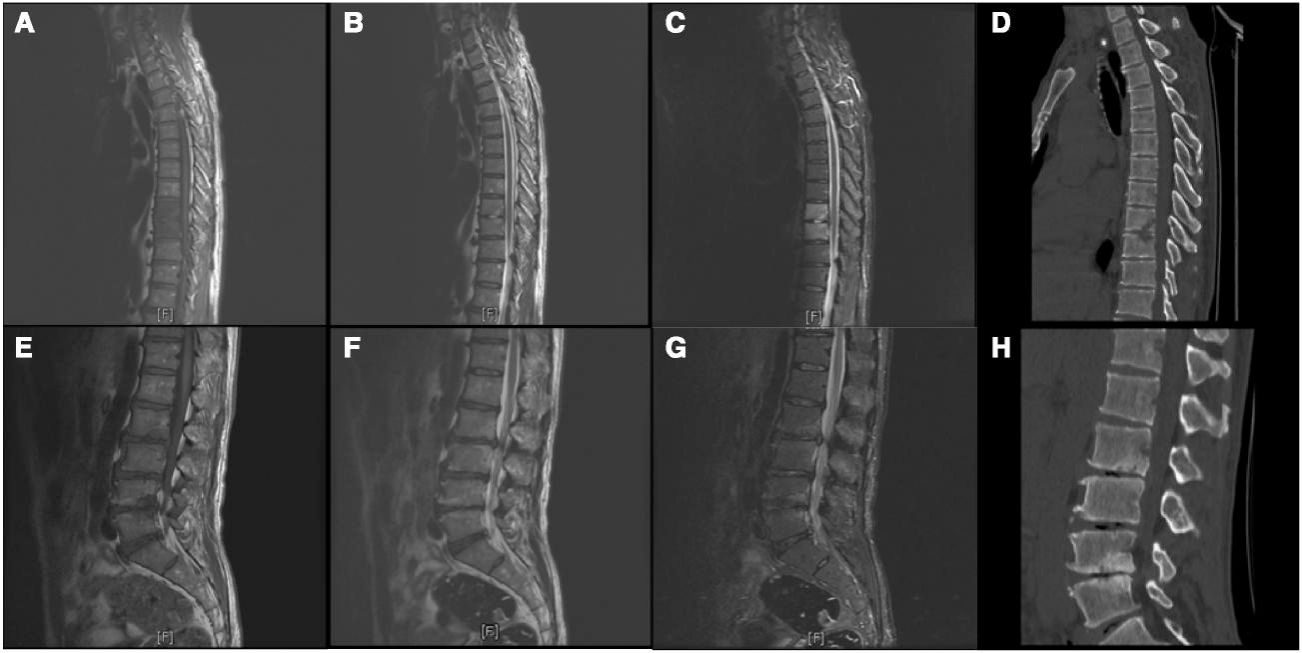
Magnetic resonance (MR) imaging and computed tomographic (CT) results on a 49-year-old man with spinal brucellosis. Sagittal T1-weighted (*A, E*) and T2-weighted (*B, F*) MR images show T9, T10, L2, L3, L4, and L5 vertebral body hypointensity and slight hyperintensity, respectively. *C, G,* T2-weighted fat-suppression images display hyperintensity in the infected vertebrae. *D, H,* CT sagittal reconstruction shows T9, T10, L2, L3, L4, and L5 vertebral body destruction and intervertebral space narrowing caused by infection.
